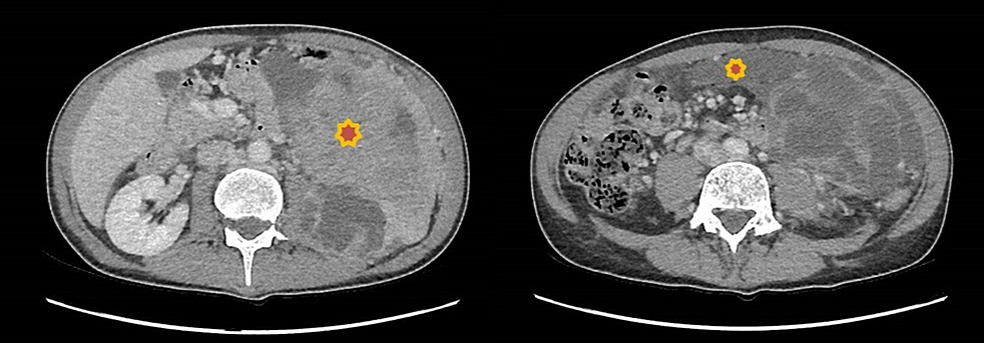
Preoperative abdominal CT scan The images show left retroperitoneal leiomyosarcoma (left) and hemoperitoneum (right) CT: computed tomography
